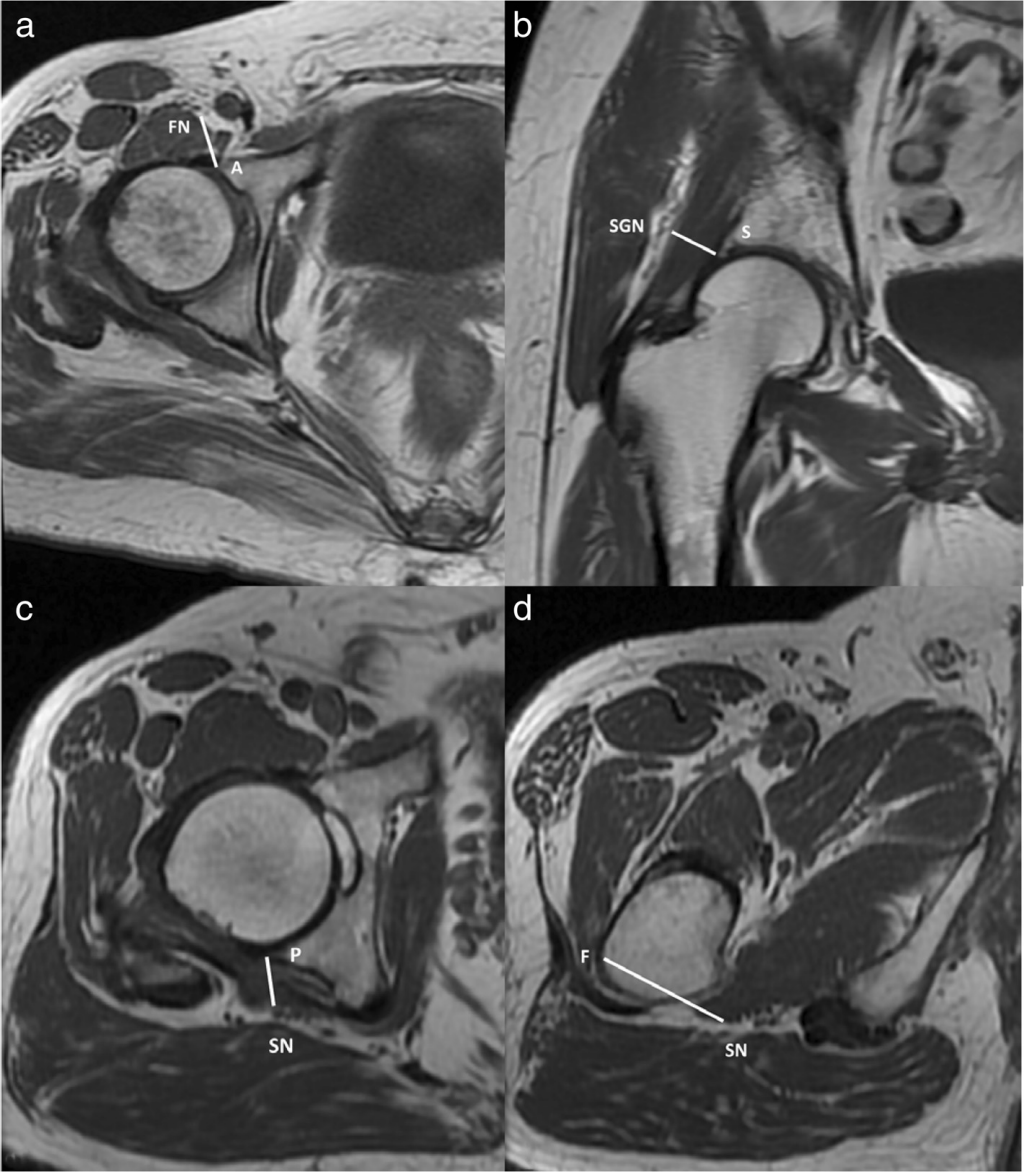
Magnetic resonance images demonstrating the views used for measuring distances from bony landmarks to adjacent nerves: a anterior acetabular rim (*A*) to the femoral nerve (*FN*); b superior acetabular rim (*S*) to the superior gluteal nerve (*SGN*); c posterior acetabular rim (*P*) to the sciatic nerve (*SN*); d greater trochanter (*G*) to the sciatic nerve (*SN*)
